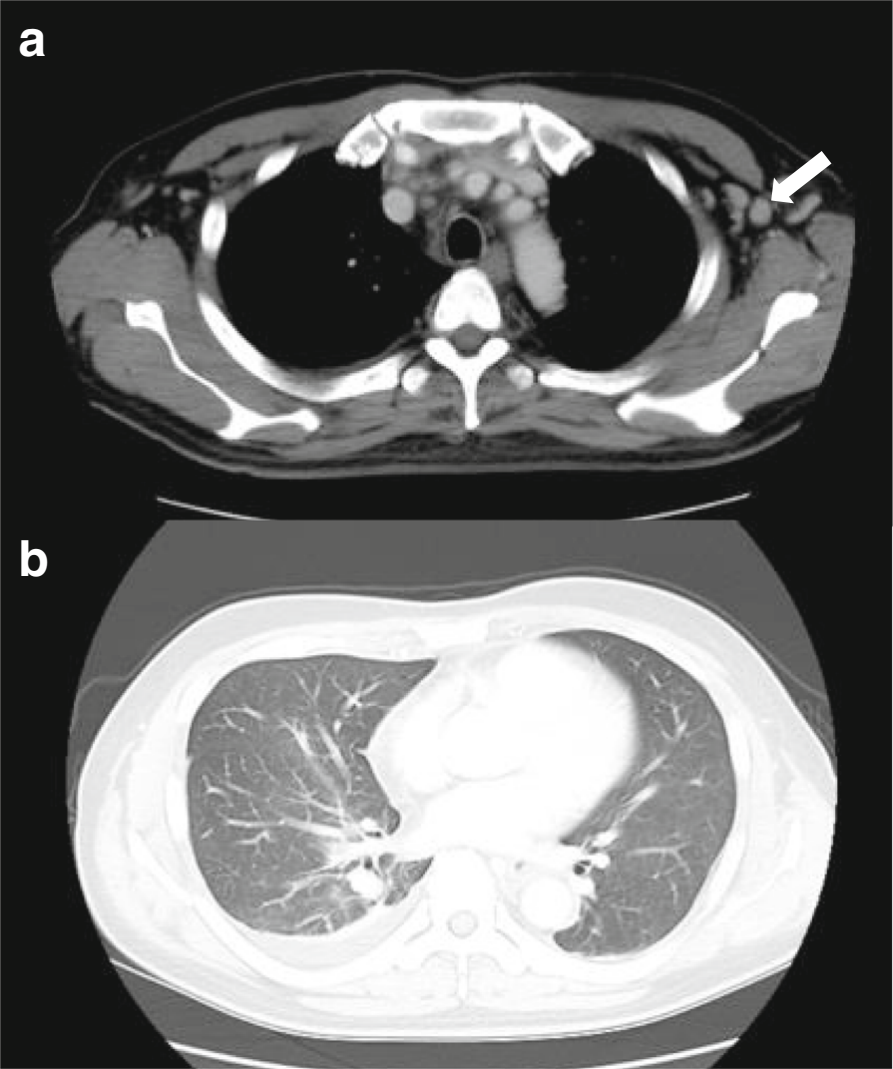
Images of the second contrasted computed tomography scan. a Left axillary lymphadenopathy (15 mm) (*arrow*). b Pleural effusion
